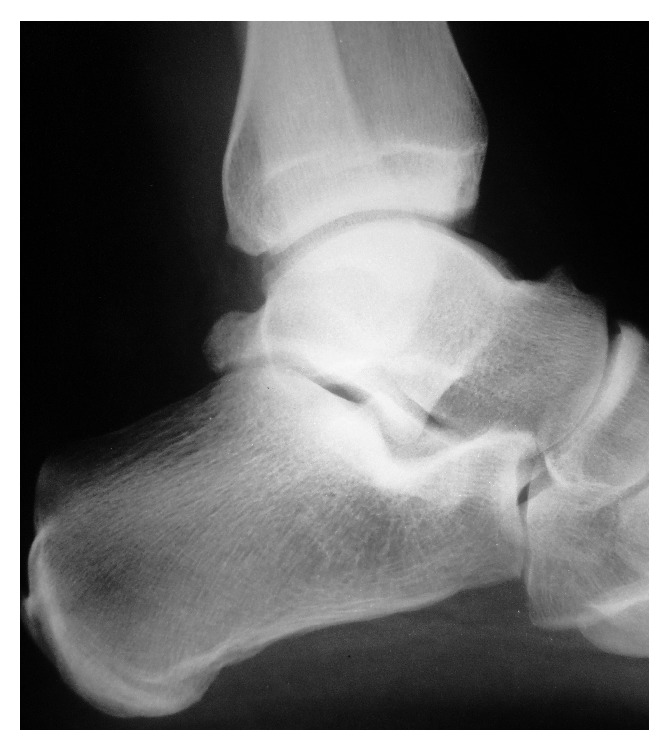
Lateral X-ray. Prominent posterior talar process.
